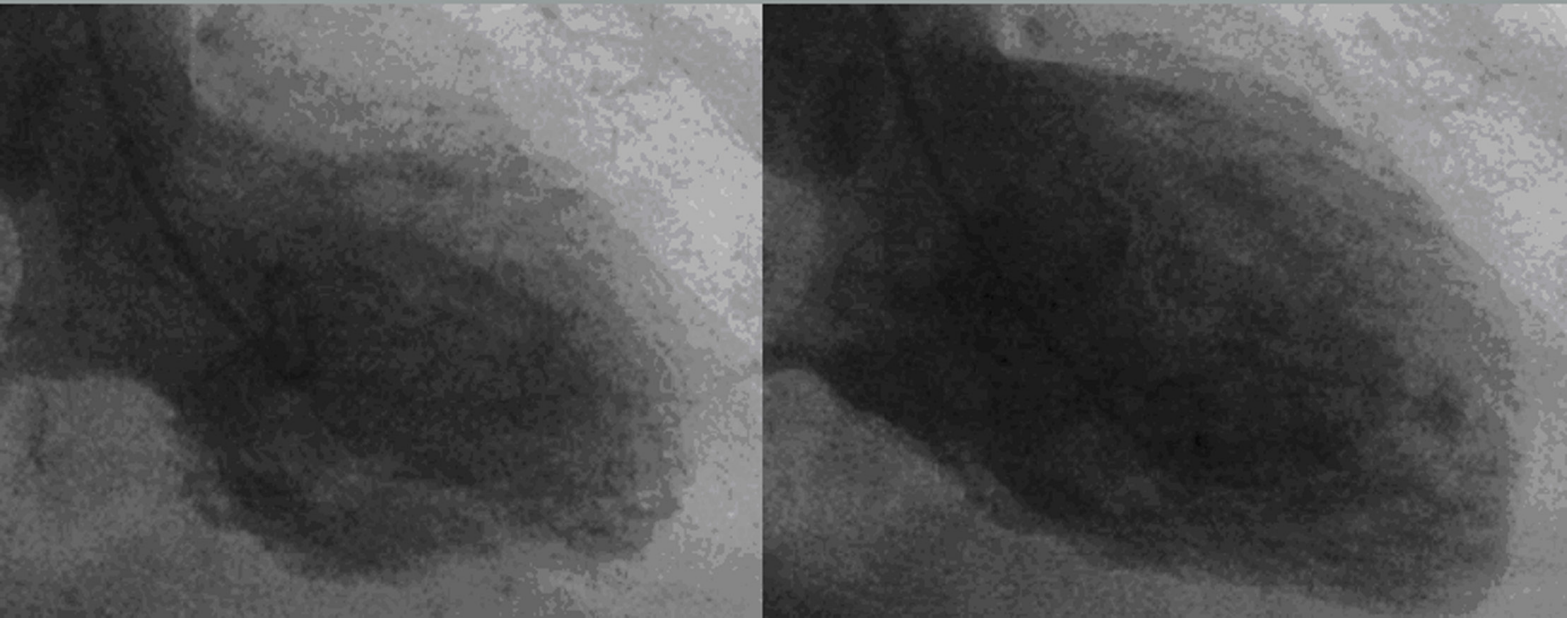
Systole and diastole during left ventriculography showing severe hyokinesis of the apical two thirds of the ventricle and preservation of systolic function in the basal segments.
